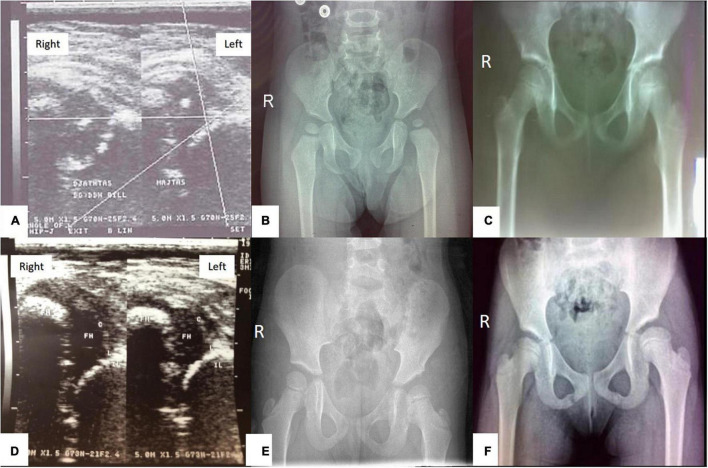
A baby girl with left hip dislocation undergoing closed reduction under analgesia and sedation (A–C), and a baby girl with bilateral hip dislocation undergoing closed reduction under general anesthesia (D–F): (A) Ultrasound examination of the hips at the age of 4.9 months showing left hip dislocation type IIIb according to Graf’s classification; (B) radiography of the hips at the age of 20 months, showing AVN type I of the left femoral head according to Ogden and Bucholz classification- hypoplastic epiphysis of the proximal femur; (C) radiography of the hips at the age of 7.9 years showing complete improvement of ossification of the left femoral head. (D) Ultrasound examination of the hips at the age of 3.9 months showing bilateral hip dislocation type IV according to Graf’s classification; (E) Radiography of the hips at the age of 2.4 years showing AVN type II of the left femoral head according to Ogden and Bucholz classification with overgrowth of greater trochanter into valgus, lateral metaphysis shows evidence of injury; (F) radiography of the hips at the age of 7.6 years showing AVN type II of the left femoral head according to Ogden and Bucholz classification – overgrowth of greater trochanter into valgus.
